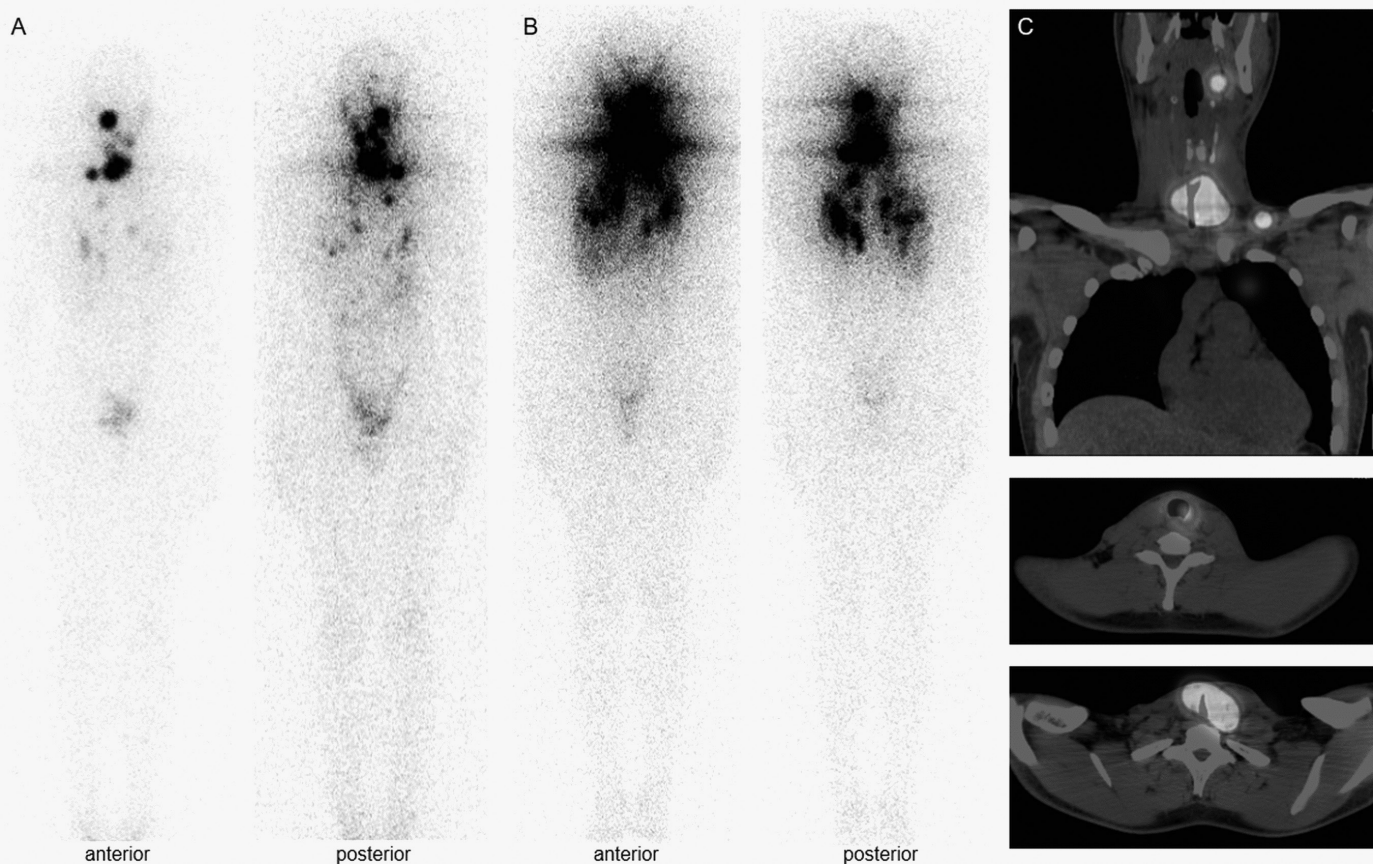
(A) Diagnostic whole-body scan after 3 months of adjuvant external beam radiotherapy. (B) Post-therapy whole-body scan revealing radioiodine-avid cervical lesions and pulmonary nodules. (C) Concomitant post-therapy single photon emission computed tomography–computed tomography (SPECT/CT) images.
